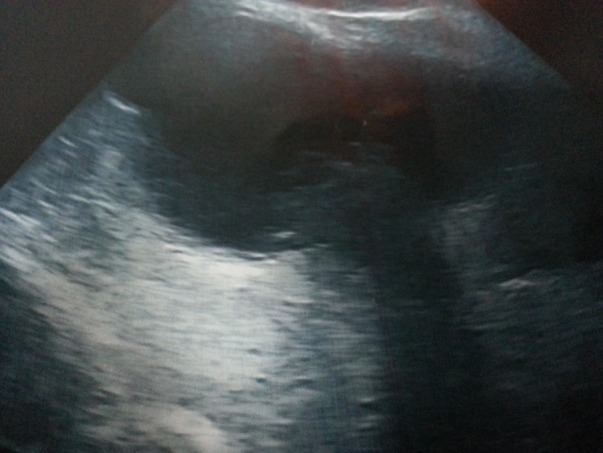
Ultrasonographic aspect of the paravesical cystic lesions
